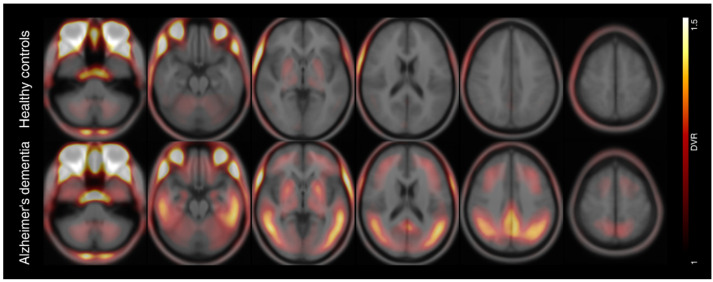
Mean parametric ^18^F-PI-2620 PET distribution volume ratio (DVR) images of healthy controls (top row) and patients with Alzheimer’s dementia (bottom row) overlayed on an averaged anatomical MRI scan in standard space of the Montreal Neurological Institute (MNI).
